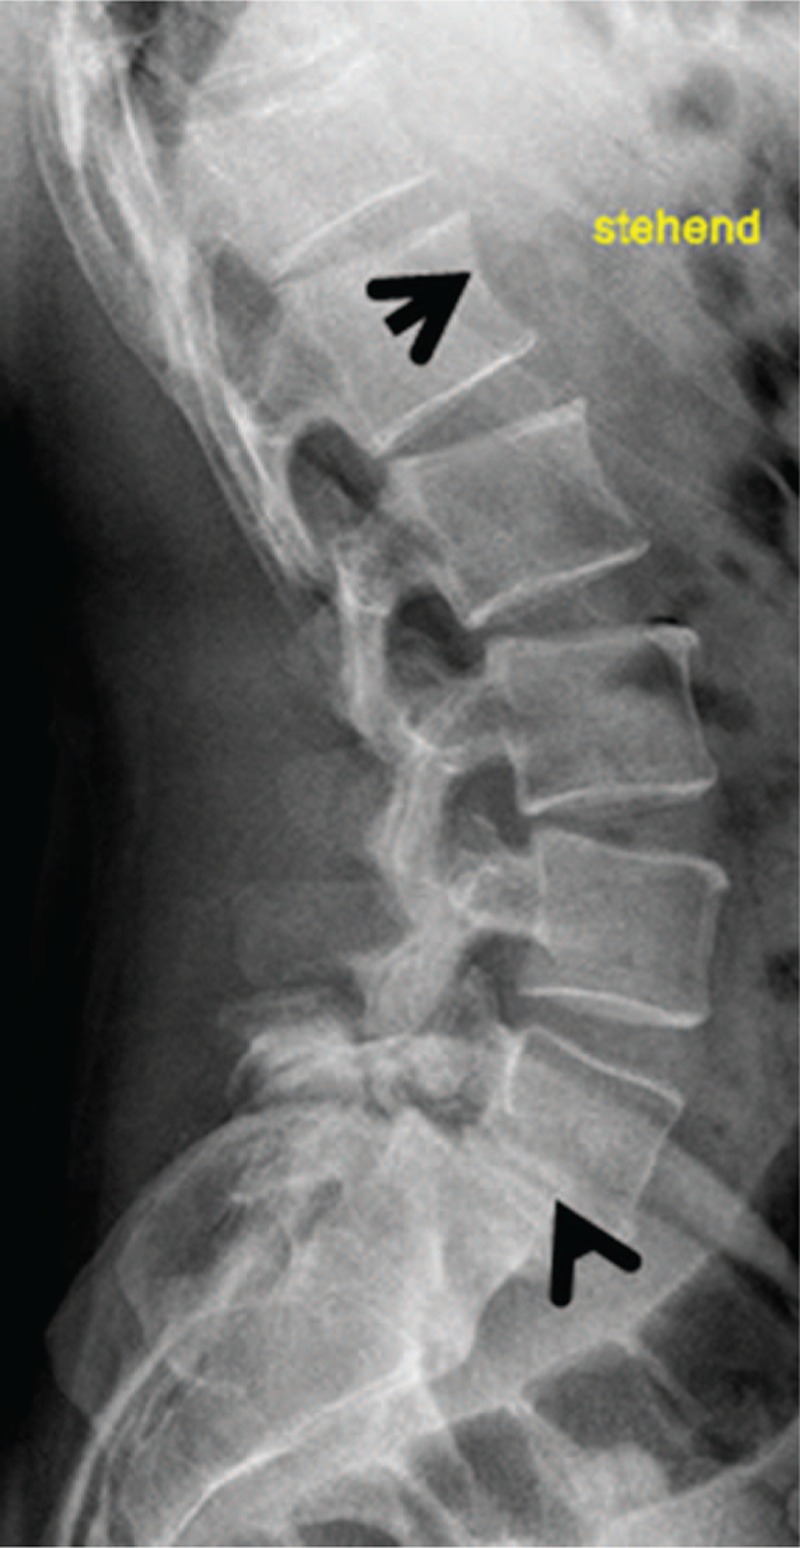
Lateral spine radiograph of the father (III,7) showed notching of the anterior end plates of the lumbar vertebrae associated with grade 2 - spondylolisthesis (forward slippage of L5/S1) because of congenital abnormality of the facets and the pars-interarticularis (arrow). Also the father is a heterozygous carrier of disease-causing mutations in the *ARSB* gene as well as in the *GNS* gene, which denotes an impaired lysosomal degradation of dermatan sulfate and heparan sulfate in the fully developed diseases MPS VI and MPS IIID.
